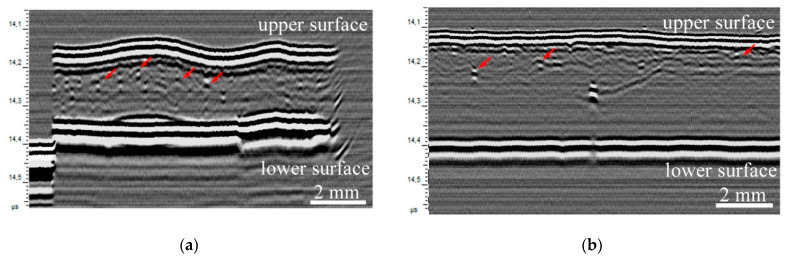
Ultrasonic vertical images of the original samples: LDPE/NR 90/10 (a), LDPE/NR 50/50 (b). Arrows are filler agglomerates.
